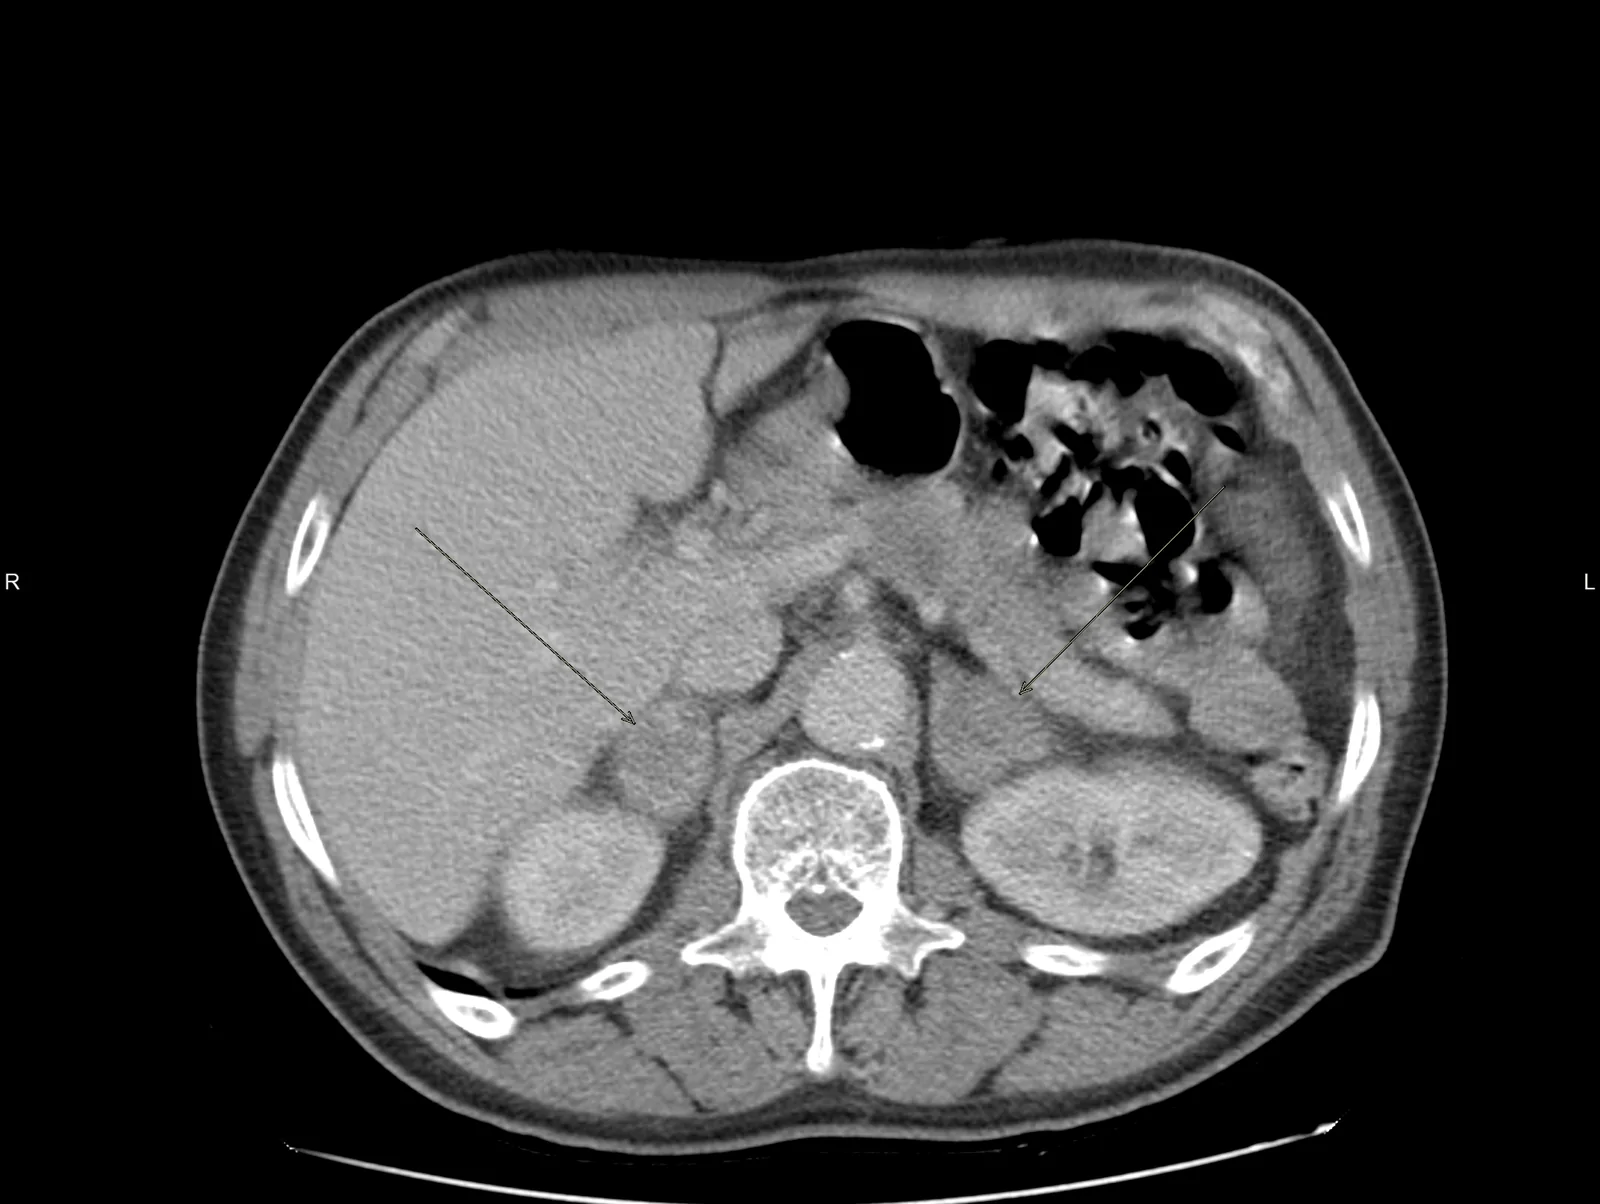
Abdominal CT demonstrating bilateral adrenal masses Axial CT of the abdomen demonstrates bilateral adrenal masses. The arrows indicate enlarged adrenal lesions measuring approximately 4.7 × 2.9 cm on the left and 3.5 × 2.5 cm on the right. Image interpretation is limited by motion artifact during image acquisition CT: computed tomography
